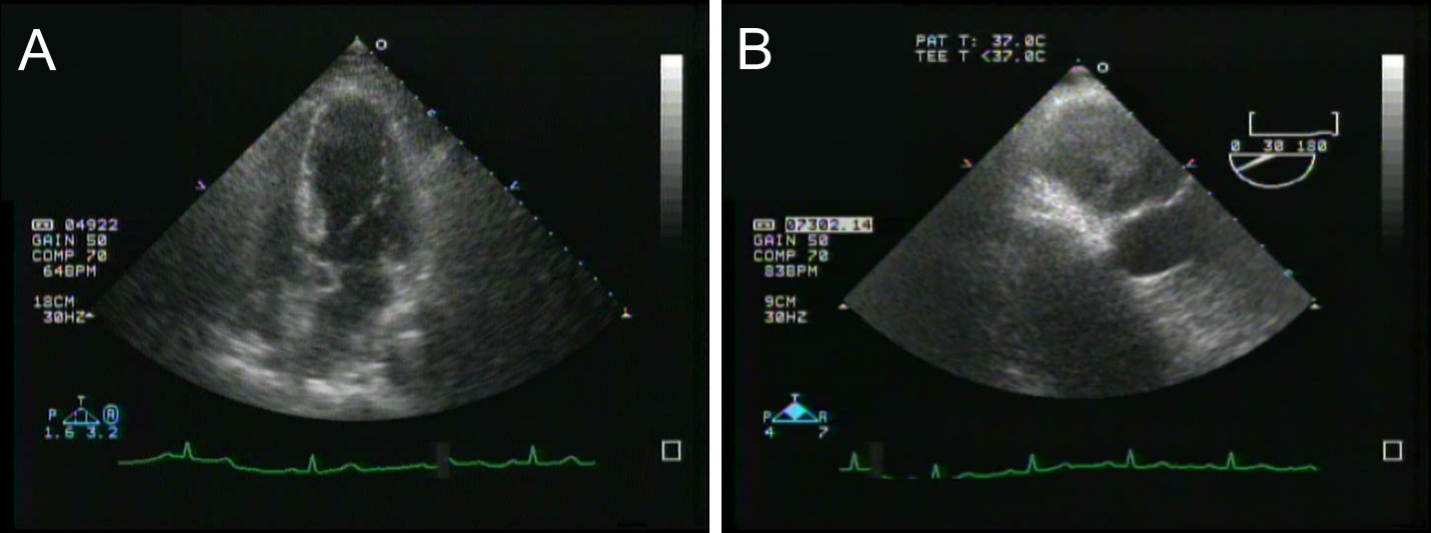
Photograph showing cardiac ultrasonography. (A) Transthoracic cardiac ultrasonography performed in our hospital showed showing a club -shaped tumor of 34 mm in diameter inside the left atrial cavity in a four-cavities tomogram. (B) Transesophageal cardiac ultrasonography showed showing a broad-based, gigantic, and multilocular tumor occupying almost the entire left atrium.
